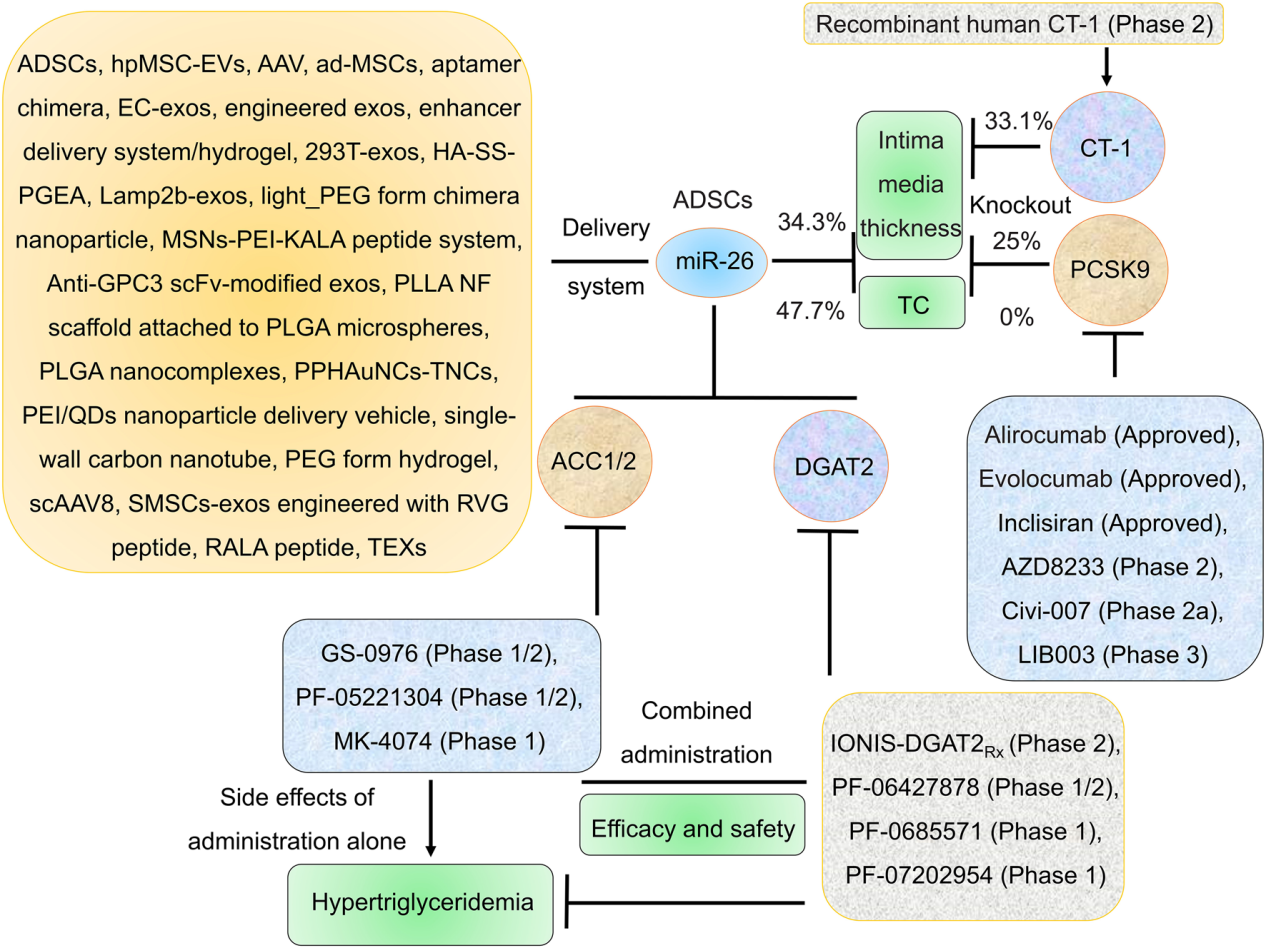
MiR-26 delivery systems and targeting ACC1/2, CT-1, DGAT2, and PCSK9 with drugs that were approved or investigated in clinical trials. ADSCs overexpressing miR-26 reduced IMT more than those with PCSK9 or CT-1 knockout. DGAT2 inhibitors can reduce the side effects of ACC1/2 inhibitors. Multiple materials have been used to deliver miR-26. *ACC1* acetyl-CoA-carboxylase 1, *CT-1* cardiotrophin-1, *PCSK9* Proprotein convertase subtilisin/kexin type 9
